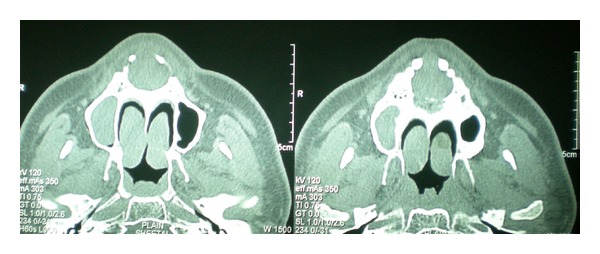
Axial sections of CT scan showing the well-defined round radiolucency.
